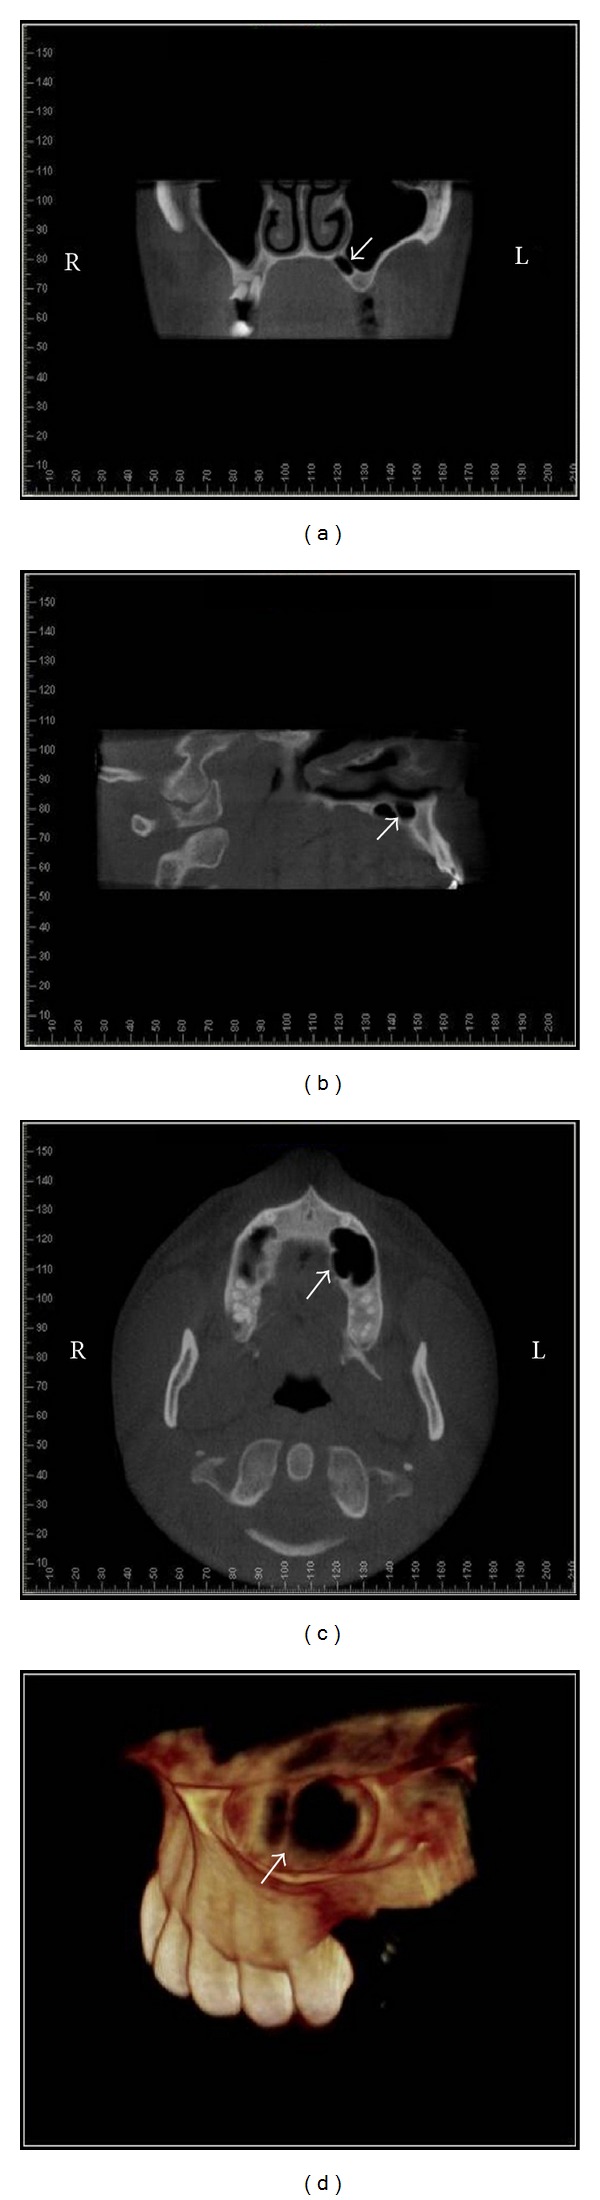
CBCT images: coronal (a), sagittal (b), and axial (c) slices and 3D reconstruction (d) in which the septum and palatine extension maxillary sinus are clearly seen on left side (arrows). R: right side and L: left side.
